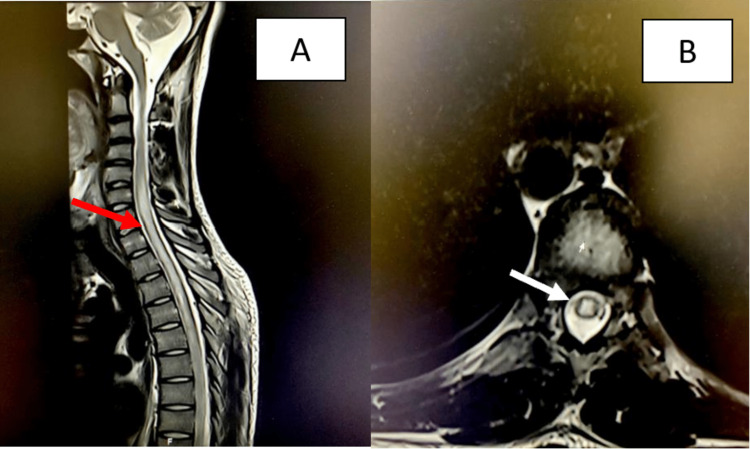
MRI of the spine Cervical and thoracic sagittal (A) and axial (B) T2-weighted MRI shows longitudinal extensive myelitis located centrally within the spinal cord from C5 to T10 intramedullary lesions as indicated by the red and white arrows.
